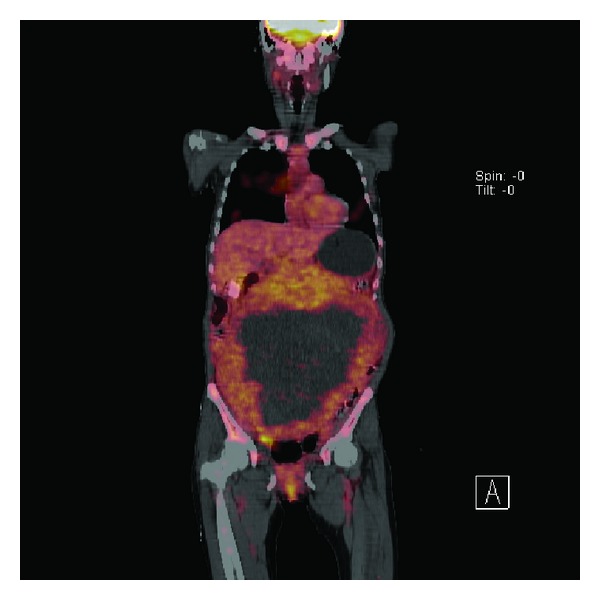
F-18 fludeoxyglucose (FDG) positron emission tomography/computed tomography (PET/CT) images.
